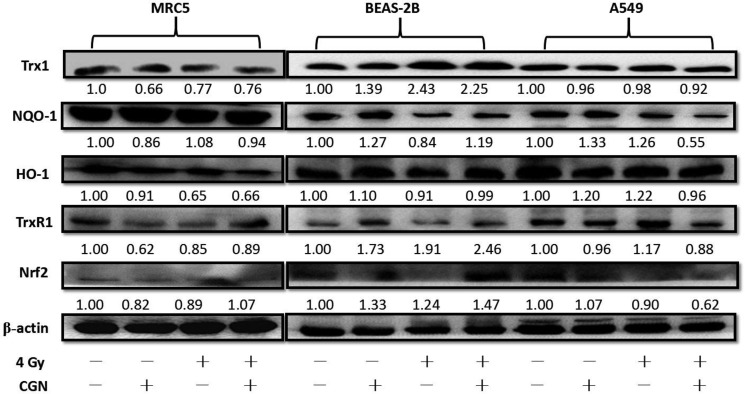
Down-regulation of the antioxidant defense system in cancer cells and up-regulation in normal cells after pretreatment with CGN and/or irradiation The antioxidant molecules expression were measured by western blotting in normal cells (MRC5 and BEAS-2B) and cancer cells (A549) at 24 h after pretreatment with or without 1 μM drugs for 24 h and then followed by 0 or 4 Gy X-ray irradiation. The cells (1×10^6^ cells/dish) were seeded in 60 mm dishes for 24 h, followed by CGN treatment for another 24 h and then exposed to 4 Gy X-rays. The cells were harvested at 24 h post-irradiation. The expressions of proteins were analyzed by western blotting. All experiments were repeated independently for three times and the typical images were presented.
